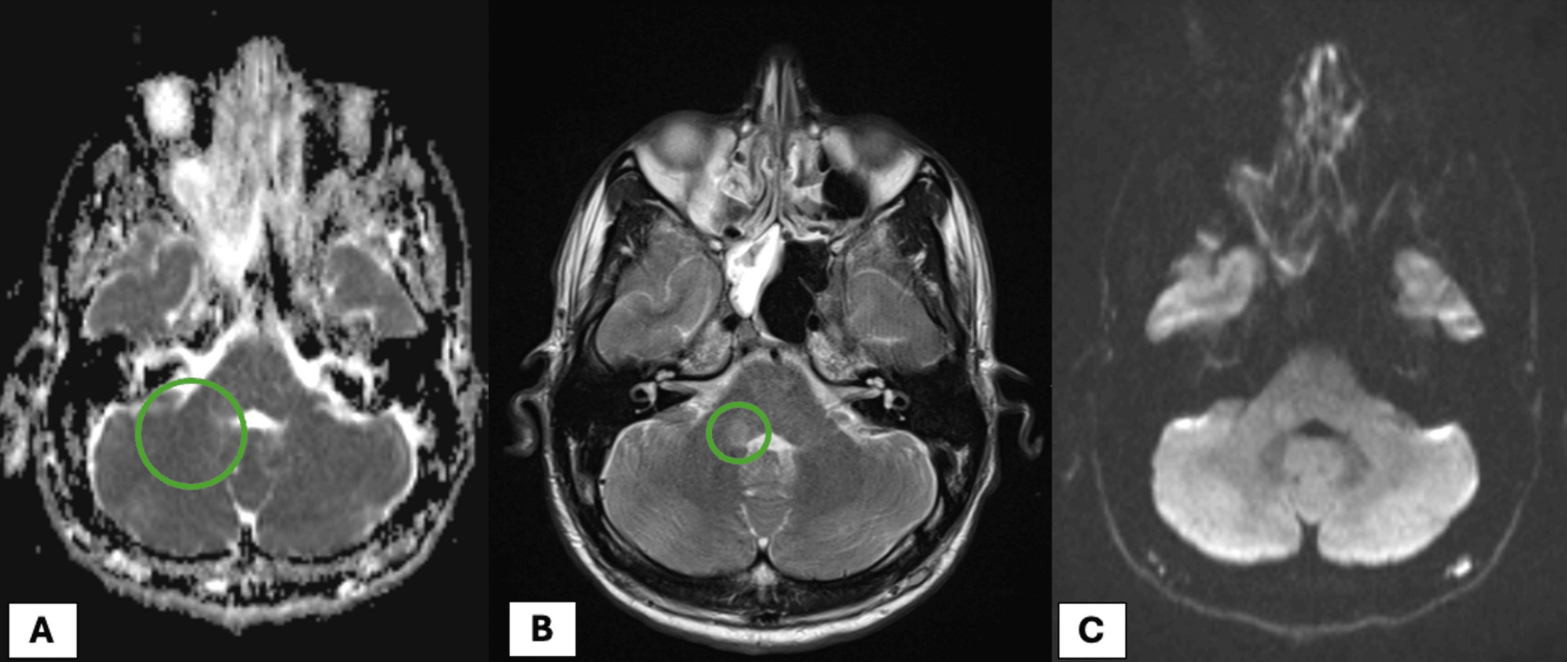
MRI head showing a focal T2 hyperintensity in the right posterior fossa involving the middle cerebellar peduncle, extending into the medulla and abutting the fourth ventricle. There is no associated enhancement or restricted diffusion. Findings are consistent with an inflammatory process.
